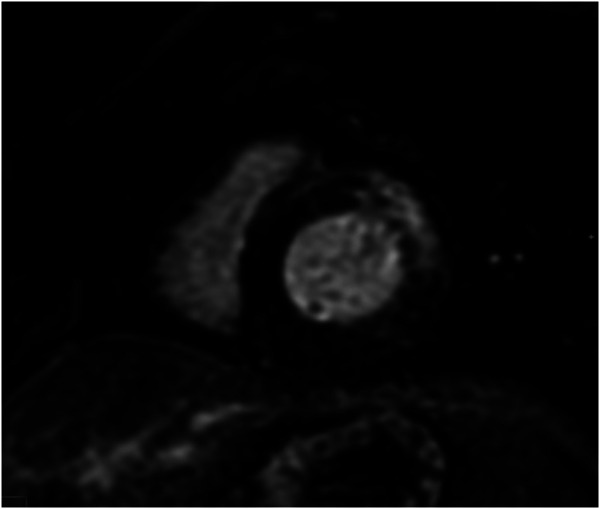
Serial cardiac magnetic resonance imaging demonstrating extensive subepicardial delayed enhancement primarily affecting the anterolateral myocardium.
